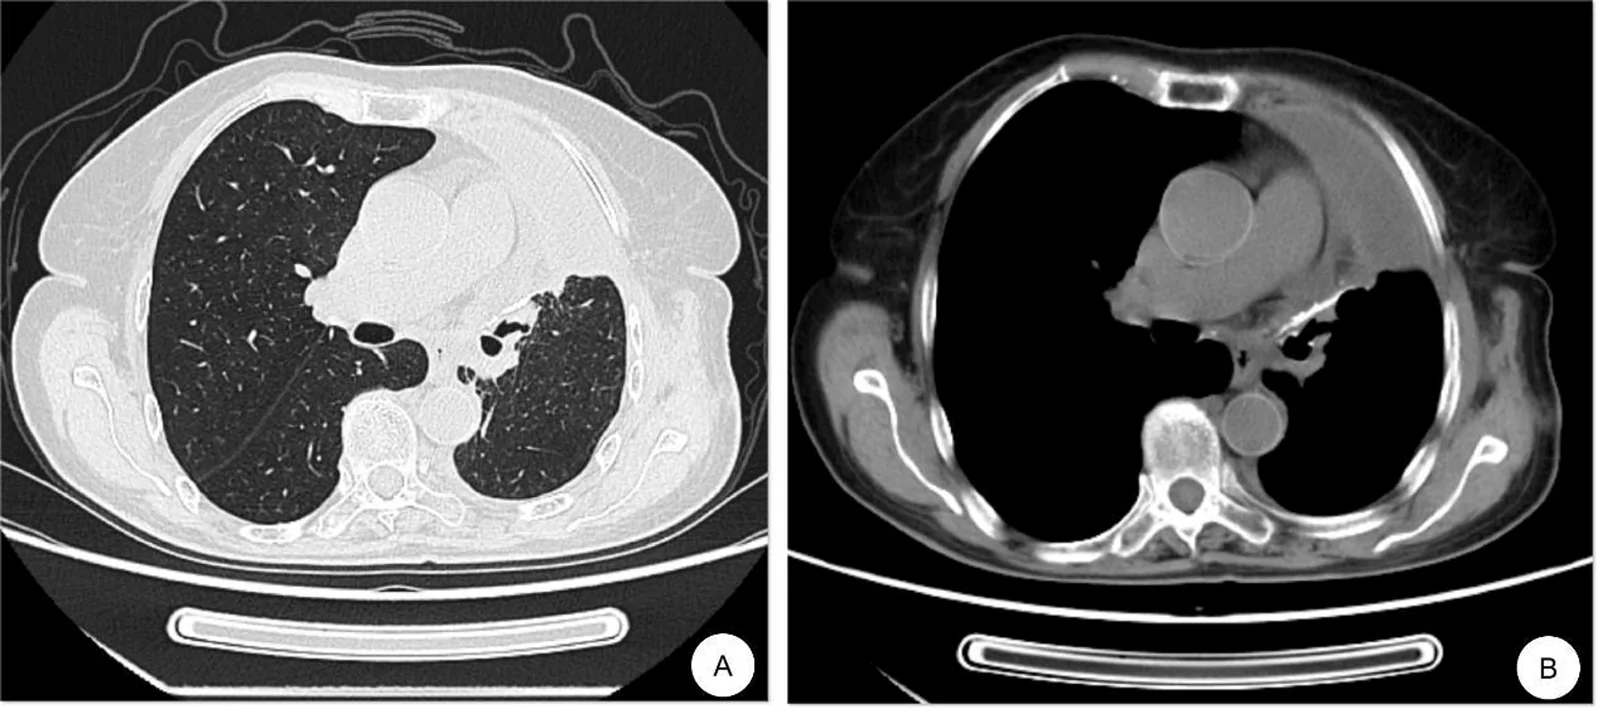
Noncontrast Chest Computed Tomography on Postoperative Day 23 After Left Upper-Lobe Sleeve Resection. (A) Lung window. (B) Mediastinal window. The left pleural air and fluid have decreased substantially compared with postoperative day 2.
